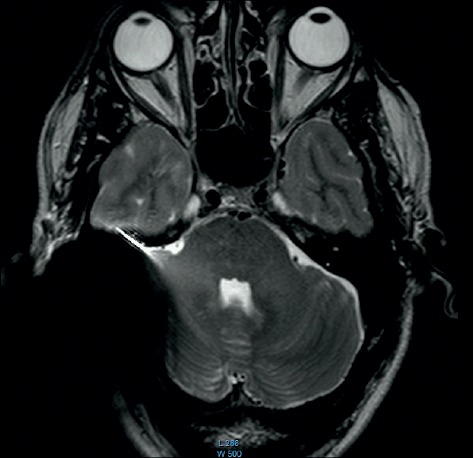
MRI pattern of the axial plain of a 1.5 Tscanning Oticon ZTI Implant.
